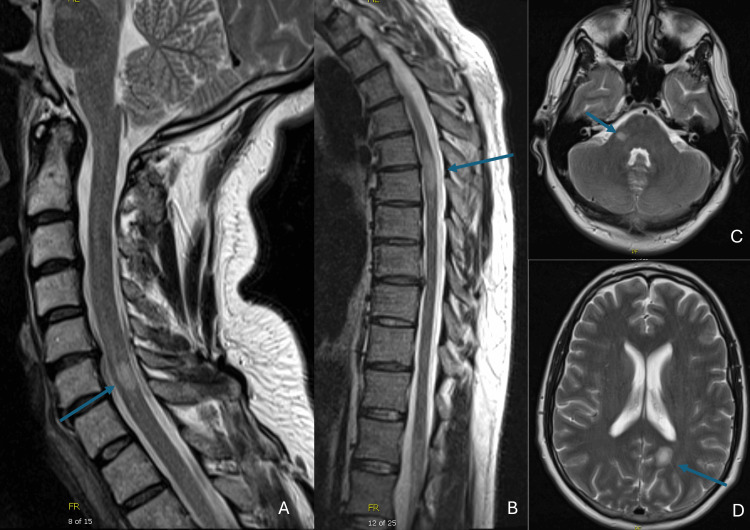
MRI T2 images showing demyelinating lesions (arrows) of the cervical cord at C6-C7 (A), thoracic cord at T5-T6 (B), the right brachium pontis (C), and the margin of the left corpus callosum (D)
